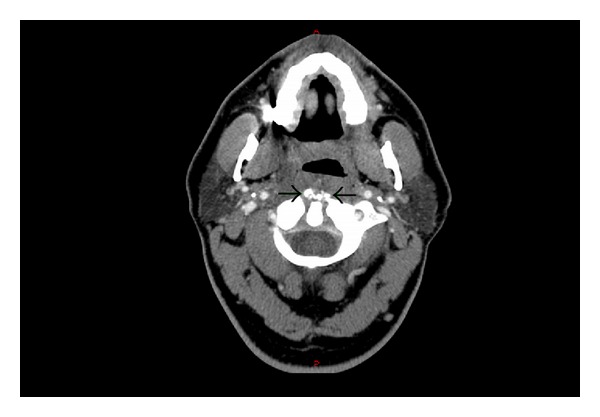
Axial CT of the neck with IV contrast on hospital day #4. Calcifications of the longus coli tendon are apparent (arrows).
